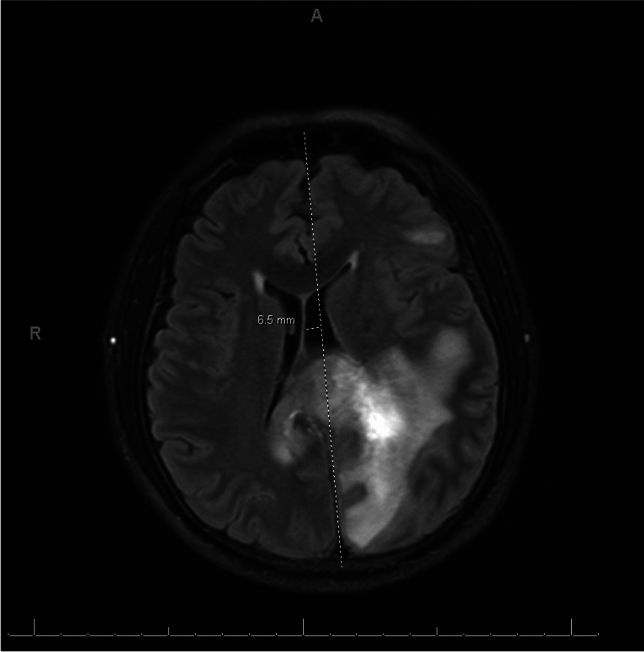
Mid-hospitalization axial MRI brain with plus without contrast displaying evolving hemorrhage within the FLAIR hyperintense enhancing mass-like lesion in the left parietal lobe crossing over the splenium of the corpus callosum with mass effect and midline rightward shift of almost 7 mm
